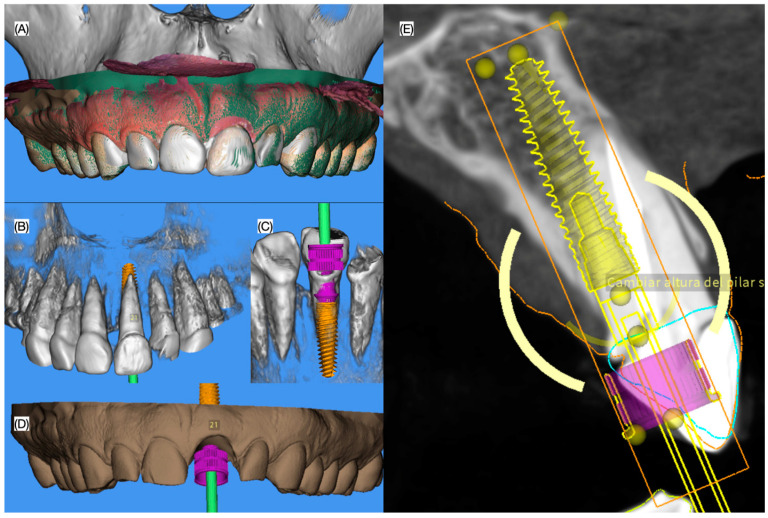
Three-dimensional planning of the implant surgery. (A) intra-oral scan overlaying the CBCT image, (B) frontal and (C) lingual view of the implant planning on the tooth area, (D) frontal view of the implant position without the existing tooth and (E) interproximal view of the implant planning.
